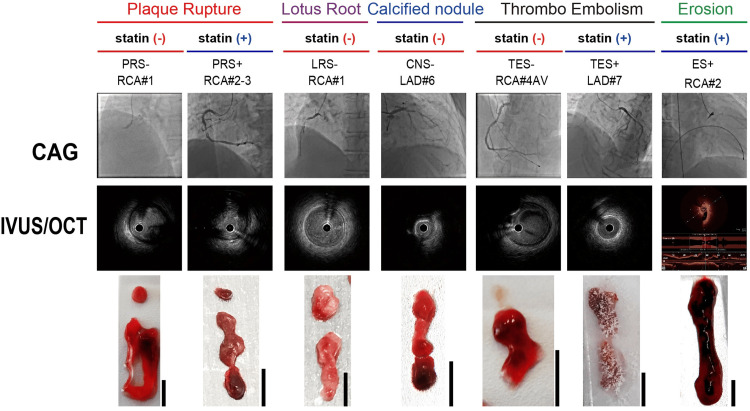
CAG, IVUS, and macroscopic classification of aspirated coronary thrombi Representative images of the culprit lesions obtained using CAG and IVUS, alongside macroscopic classifications of the aspirated coronary thrombi. Macroscopic images correspond to five thrombus types: PR, LR, CN, TE, and E. Scale bars (5 mm) are shown in each panel. Plaque rupture (IVUS): Fibrous cap disruption with a cavity communicating with the lumen. Lotus-root-like lesion (IVUS): Multiple intraluminal channels separated by septa, consistent with recanalized organized thrombus. Calcified nodule (IVUS): Protruding, irregular calcified mass extending into the lumen with acoustic shadowing. Plaque erosion (OCT): Attached thrombus over an intact fibrous cap without evidence of cap disruption. Thromboembolism (TE): Culprit-lumen thrombus without intravascular imaging evidence of an underlying atherosclerotic lesion, supported by angiographic and/or clinical features suggestive of coronary embolism. CAG: coronary angiography, IVUS: intravascular ultrasound, PR: plaque rupture, LR: lotus-root-like lesion, CN: calcified nodule, TE: thromboembolism, E: plaque erosion
